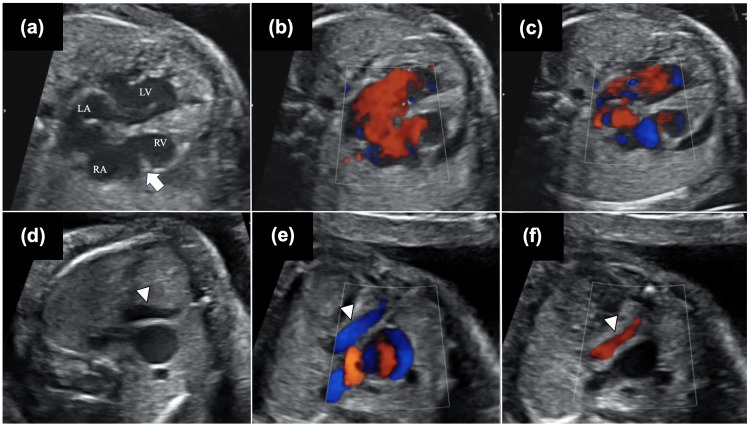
Fetal echocardiogram Four-chamber view from the fetal echocardiogram. (a) The tricuspid valve was not functioning (arrow), and the right ventricle was hypoplastic, with partial wall thinning and the morphology of Uhl's disease. (b, c) The right atrial-ventricular blood flow was to and fro (Figure 1b was a systolic phase, and Figure 1c was a diastolic phase). (d-f) The coronary artery fistula (arrowhead), misdiagnosed as the main pulmonary artery before birth, was located anterior to the aorta, displaying a to-and-fro flow on color flow mapping. RA, right atrium; RV, right ventricle; LA, left atrium; LV, left ventricle
